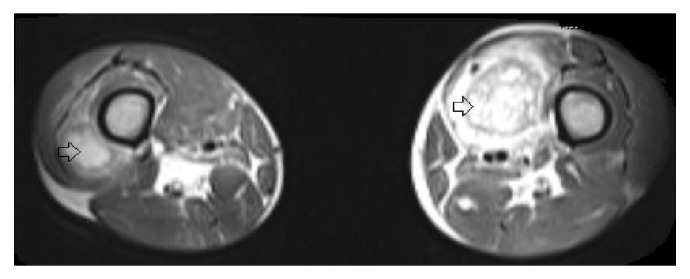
MRI of bilateral thighs showing contrast-enhancing metastatic skeletal lesions (arrows).
